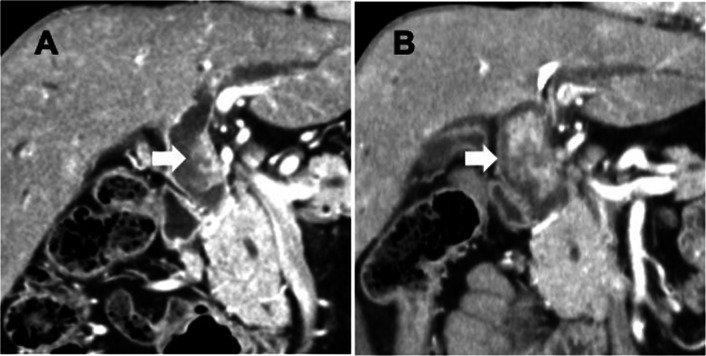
Computed tomography images at presentation showed a nodular mass at the common hepatic duct (A). Imaging before surgery showed rapid tumor growth (B)
